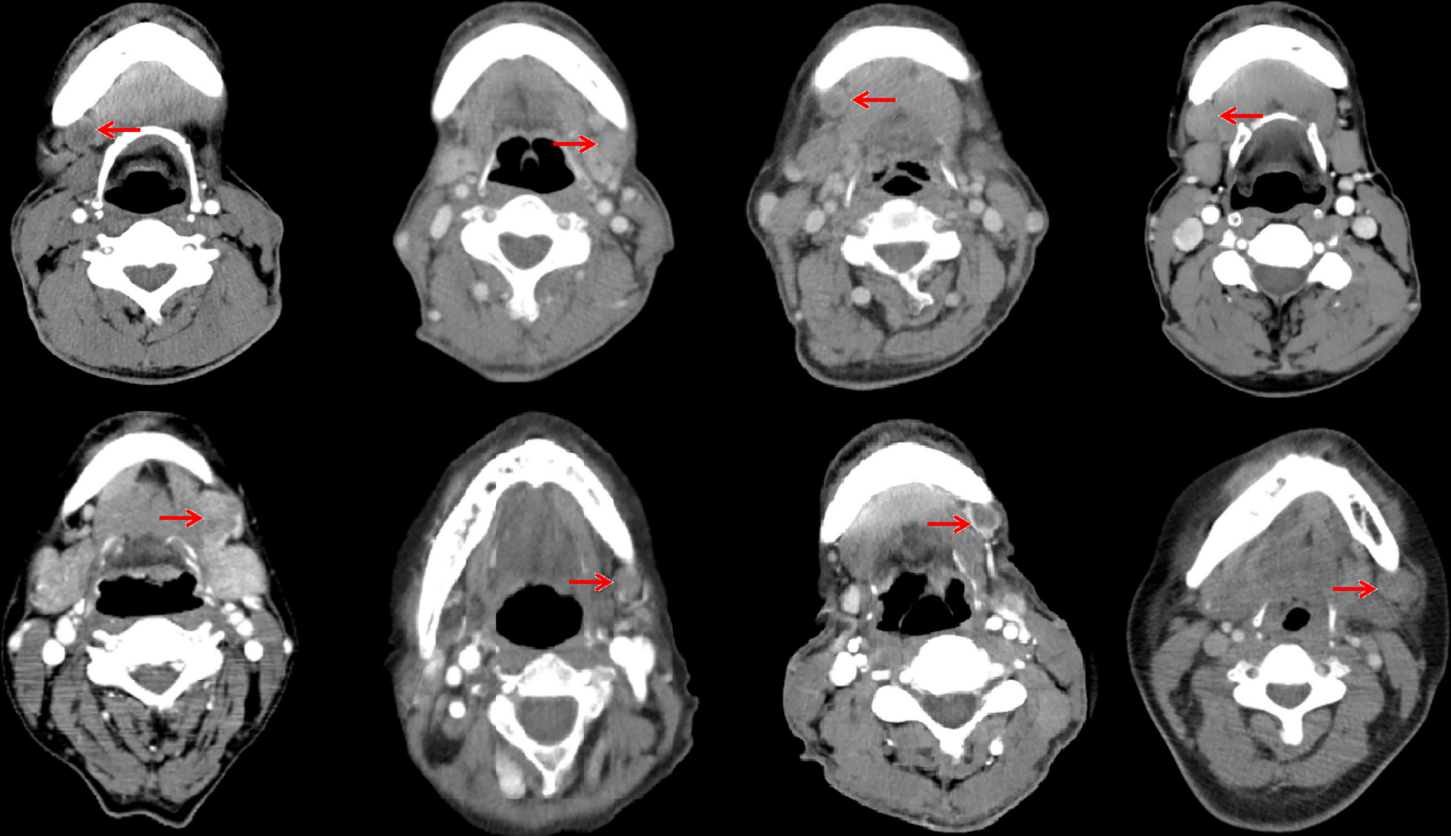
Computed tomography axial images from patients with lymphadenopathy in level IB (red arrow, lymphadenopathy in level IB).
